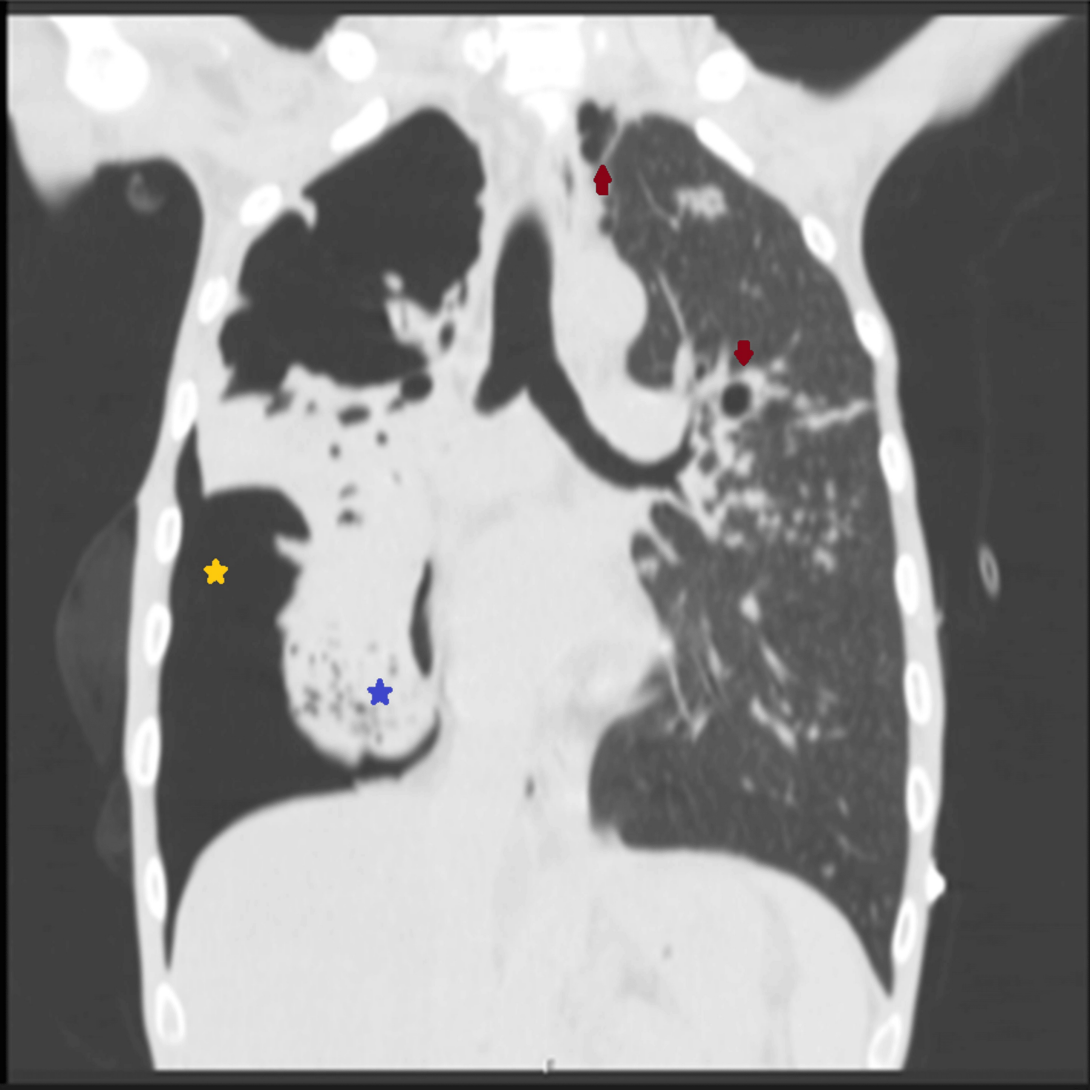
Coronal chest CT scan image Coronal image showing an extensive right-sided pneumothorax (yellow star) with lung atelectasis (blue star) and small cavitations visible in the left lung (red arrows).
